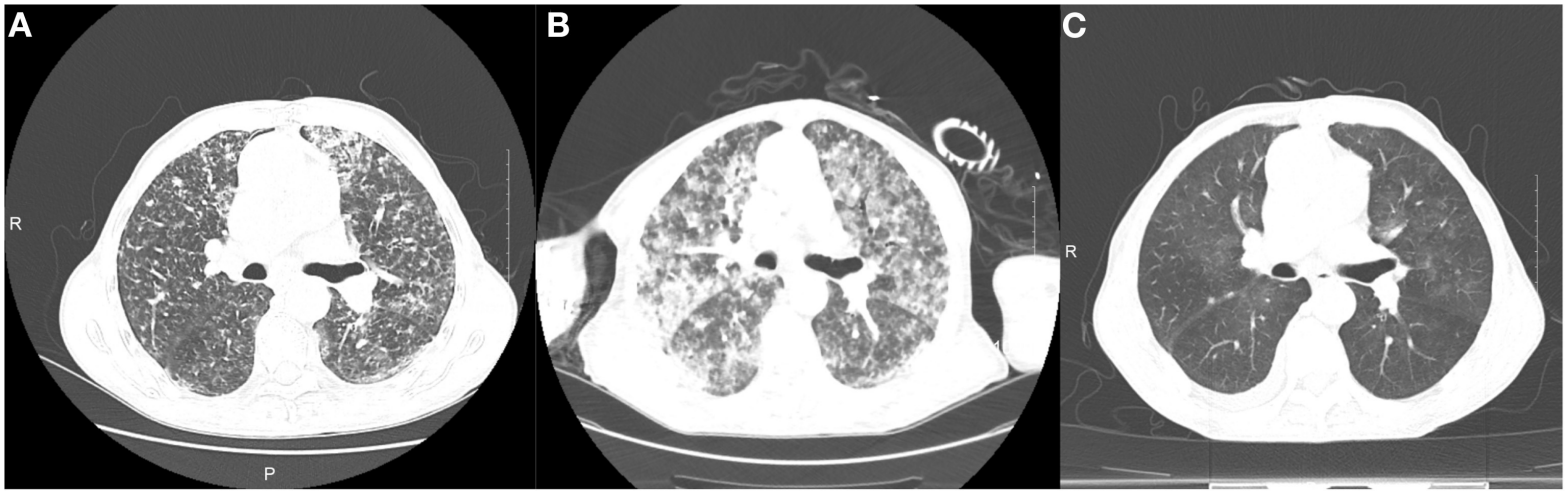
Computed tomography findings. Computed tomography images were respectively taken on admission (A), after intravenous piperacillin tazobactam (B), and before discharge (C).
